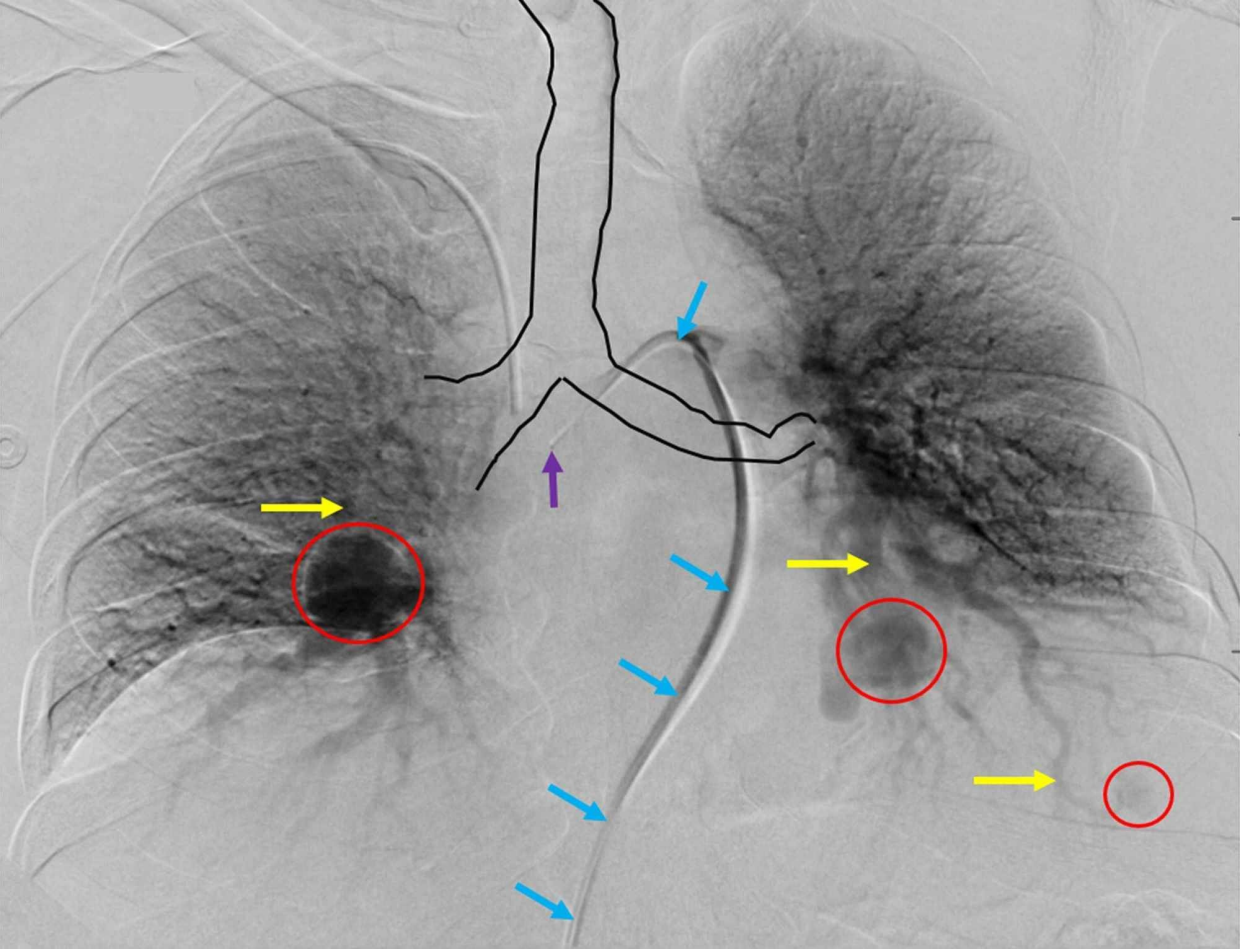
Pulmonary Arteriogram Showing Three Separate AVMs and Respective Supplying Arterial Branches The initial arteriogram from the main pulmonary artery demonstrates three separate AVMs in the medial segment of the right middle lobe, a lateral segment of the left lower lobe, and an anteromedial segment of the left lower lobe (red circles). The two larger AVMs correlated with the observed findings on the initial CT of the chest. An occult AVM (red circle, bottom right of image) was only definitively visualized on CT of the chest, after meticulous retrospective analysis. The yellow arrows demonstrate the pulmonary artery branches that supply the identified AVMs. The blue arrows show the sheath accessing the main pulmonary artery, with the tip of the wire displayed by the purple arrow. The trachea can be seen outlined in black for visual reference.
